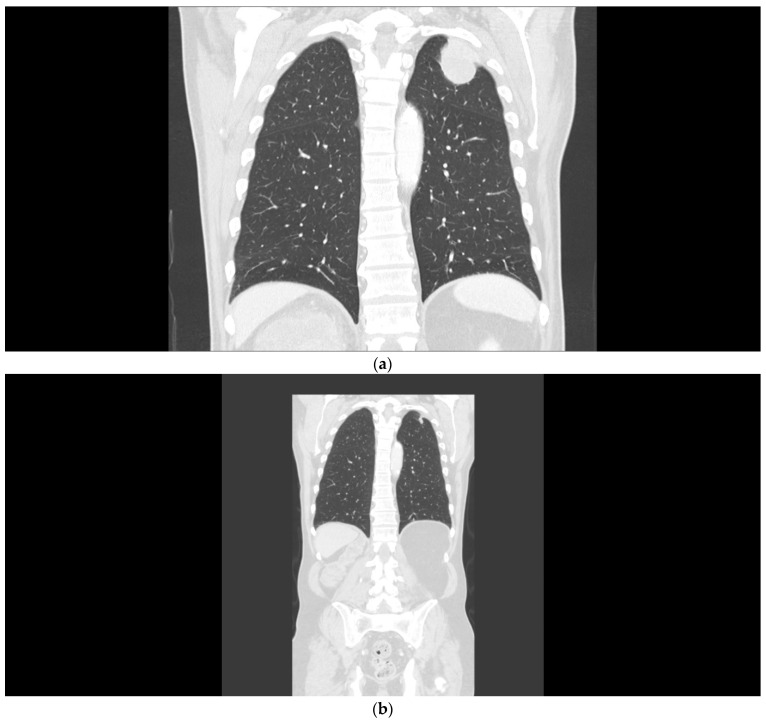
(a) Patient 1, pretreatment CT of the chest demonstrating lung metastases. (b) Patient 1, marked tumor reduction after the combination regimen of ipilimumab with nivolumab.
